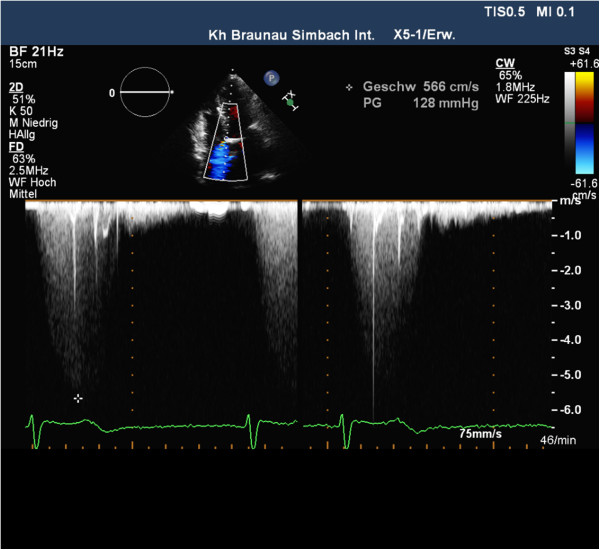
Two-dimensional color-flow Doppler echocardiogram shows blood flow during systole from the left ventricle to the right atrium through the Gerbode defect (arrows) in apical 4-chamber view.
